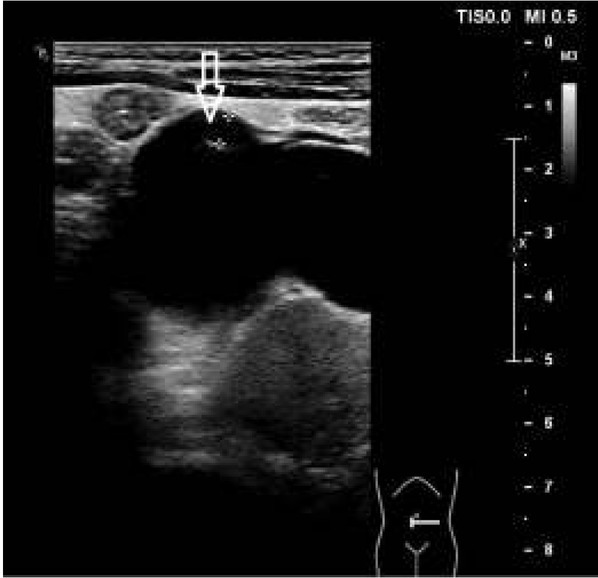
Badanie USG – widoczne wodonercze lewego układu i wysokie odejście lewego moczowodu. Fig. 1. Ultrasonography images of left collecting system hydronephrosis and high insertion of the left ureter.
